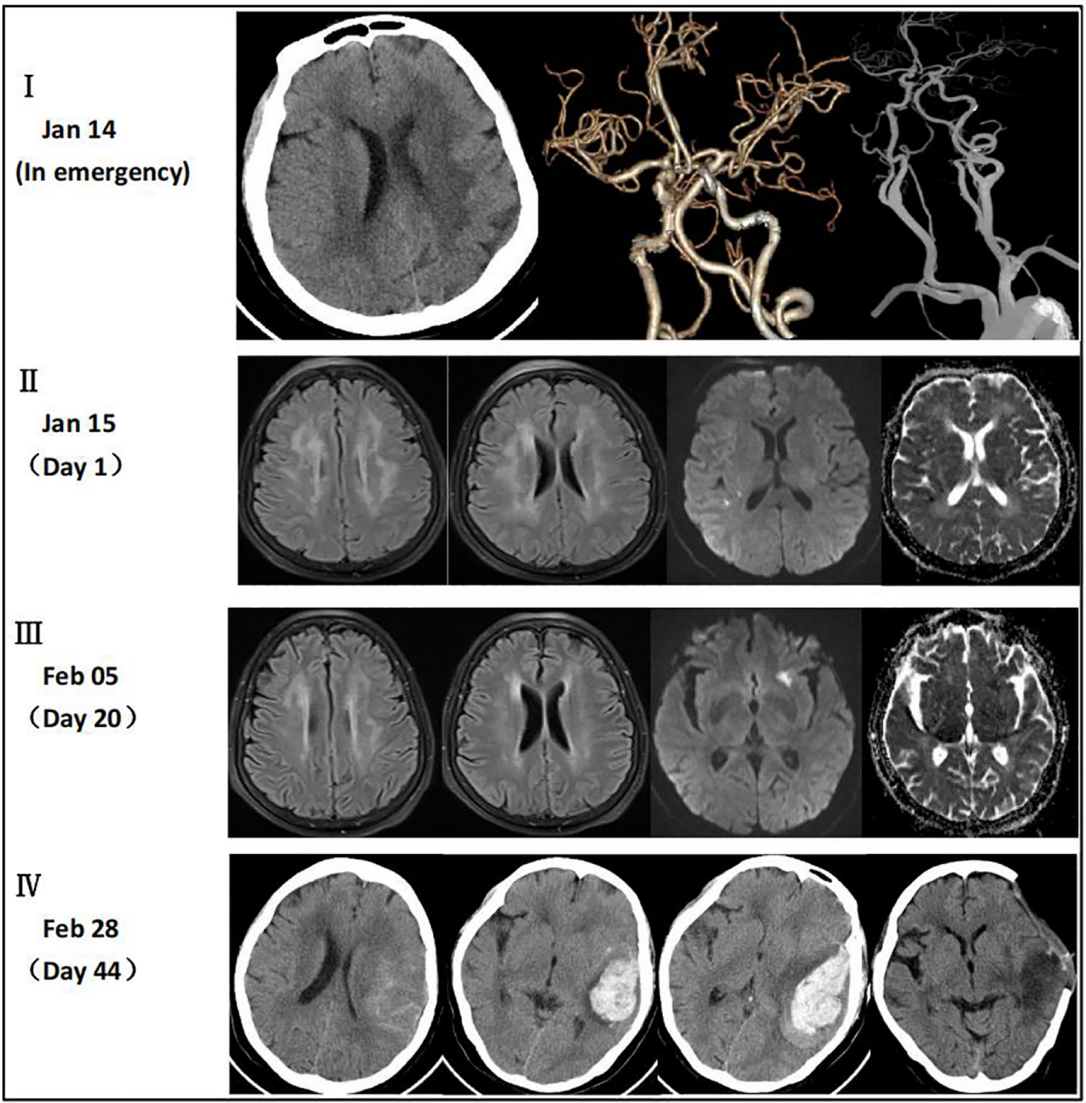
(I) CT angiography did not reveal any signs of cerebral hemorrhage or large vessel occlusion. (II) MRI showed multiple intracranial demyelination lesions and infarction only in the right temporal lobe and posterior horn of the ventricle. (III) MRI showed improvement in the intracranial demyelination lesions and a new IS in the left external capsule. (IV) CT scan indicated a HS of approximately 66 mL which was absorbed within 33 days after the operation.
